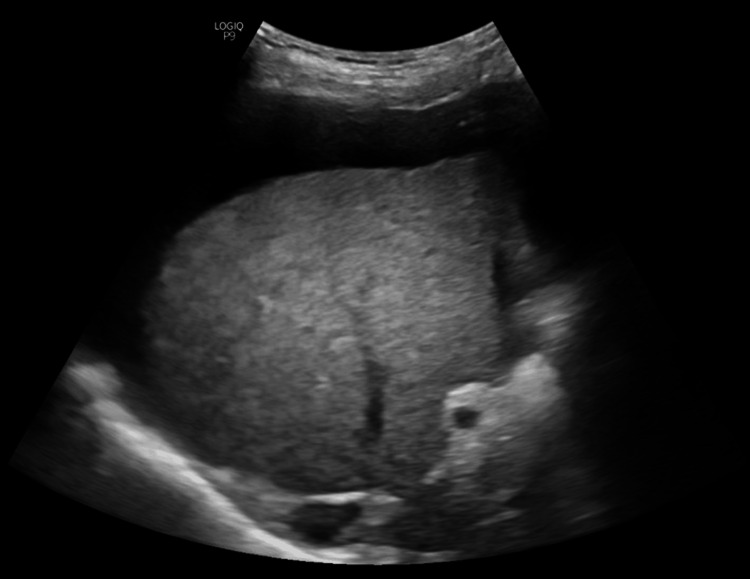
Abdominal ultrasound image of case 2. A coarsened echotexture of the liver with an irregular surface and ascites.
